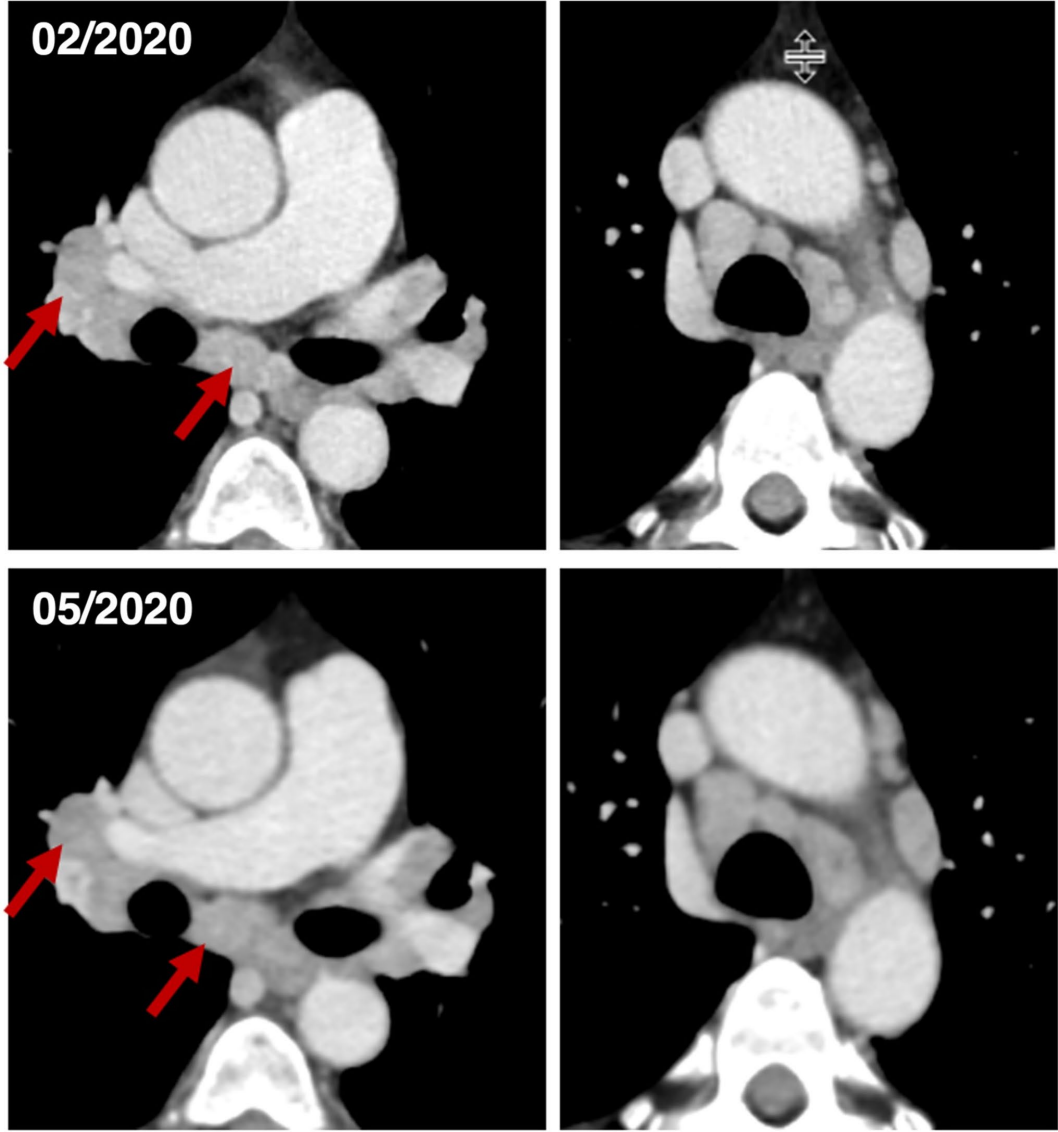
CT follow-up staging of a 65-year-old female patient three months after curative resection of a uterine leiomyosarcoma (02/2020). Another follow-up staging three months later (05/2020) showed no relevant changes. Suspicious bihilar lymph nodes proved to be epithelioid cell granuloma without necrosis compatible with a sarcoid-like reaction
